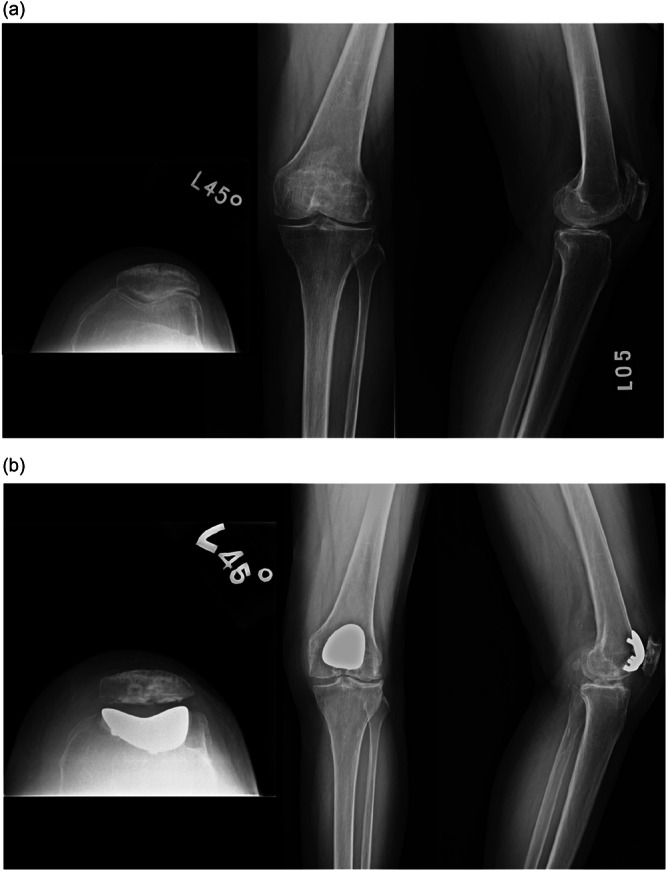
(a) A 68‐year‐old female underwent patellofemoral arthroplasty (PFA) in 2014. Preoperative plain radiographs showed grade IV patellofemoral arthritis, a small anatomical lateral distal femoral angle (aLDFA), and a lower Insall–Salvati ratio. (b) Three years later, the progression of tibiofemoral osteoarthritis led the patient to undergo total knee arthroplasty (TKA).
